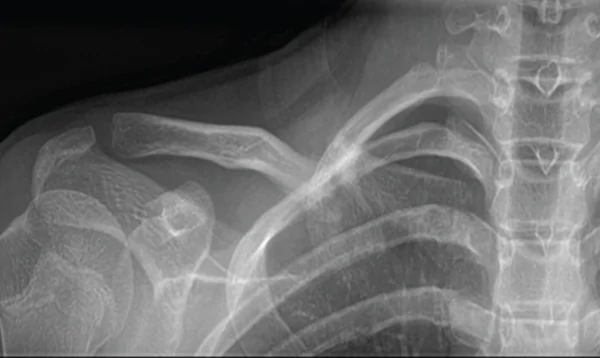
Day 59 radiographs demonstrating robust and complete callus formation with fracture consolidation prior to clearance for full return to sport.
